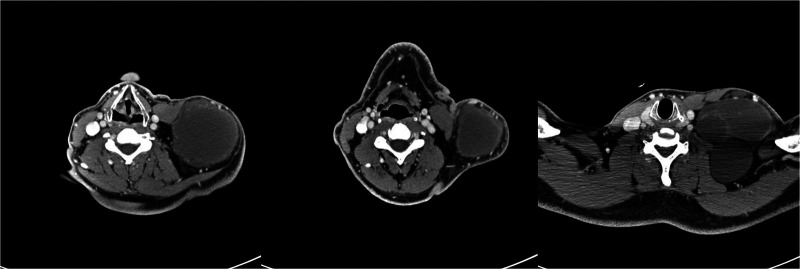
A CT scan of neck mass showing left jugular neck compression.
